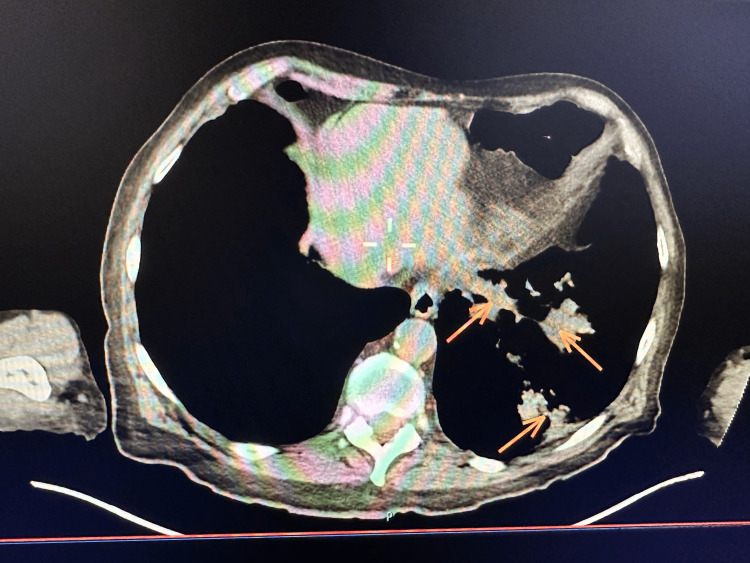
Computed tomography scan showing three pulmonary lesions in the left lower lobe of the lung.
